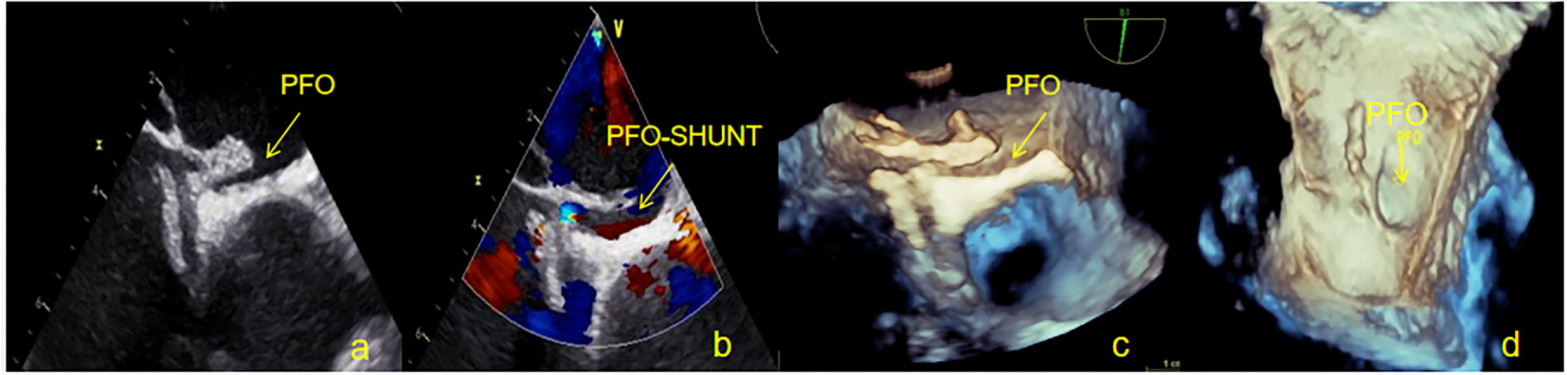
One patient with a large residual right to left shunt was observed by TEE at 12 months. (a) Two-dimensional TEE displaying the PFO gap remains open in the occluder device. (b) Doppler color flow imaging showing the left to right shunt of the PFO. (c) Three-dimensional TEE displaying the outline of the occluder. (d) Three-dimensional TEE displaying the outline of the left side occluder, where the left atrial side of the PFO remains open. TEE, Transesophageal echocardiography; PFO, Patent foramen ovale.
